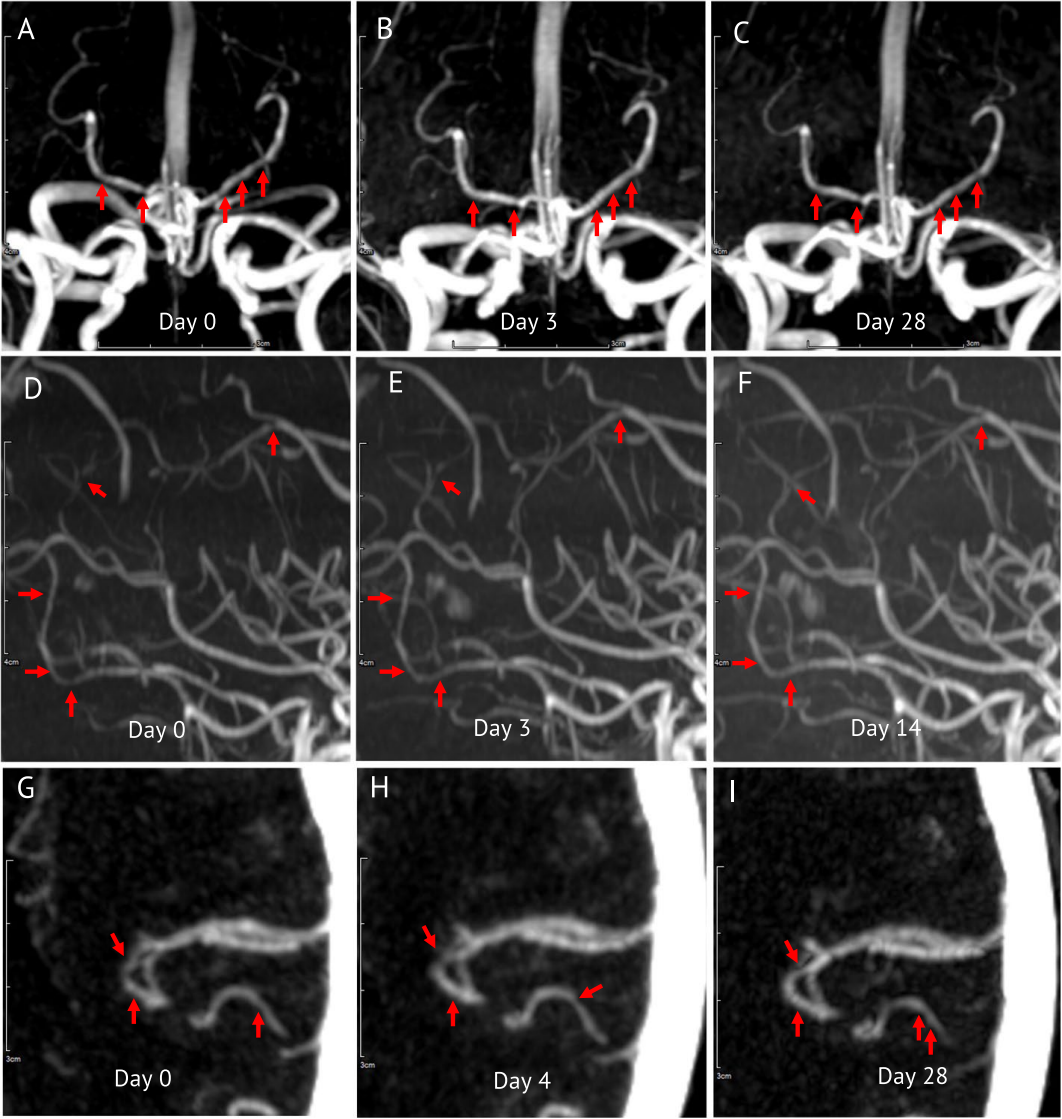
Vascular imaging features in patients with focal neurological symptoms after inactivated COVID-19 vaccination. Panel A-C, time-of-flight (TOF) magnetic resonance angiography (MRA) of the brain shows multifocal luminal irregularity and narrowing of bilateral posterior cerebral arteries (A) and subsequent imaging show reversion of the narrowing on day 3 (B) and day 28 (C). Panel D-F, TOF-MRA show multifocal narrowing of M3 segment of right middle cerebral artery (MCA) and focal narrowing of A3 segment of right anterior cerebral artery on day 0 (D) and later reversion on day 3 (E) and day 14 (F). Panel G-I, computed tomography angiography (CTA) of the brain shows focal narrowing of M2 segment of left MCA (G) and subsequent imaging show reversion of angiographic abnormality on day 4 (H) and new focal narrowing on day 28 (I)
